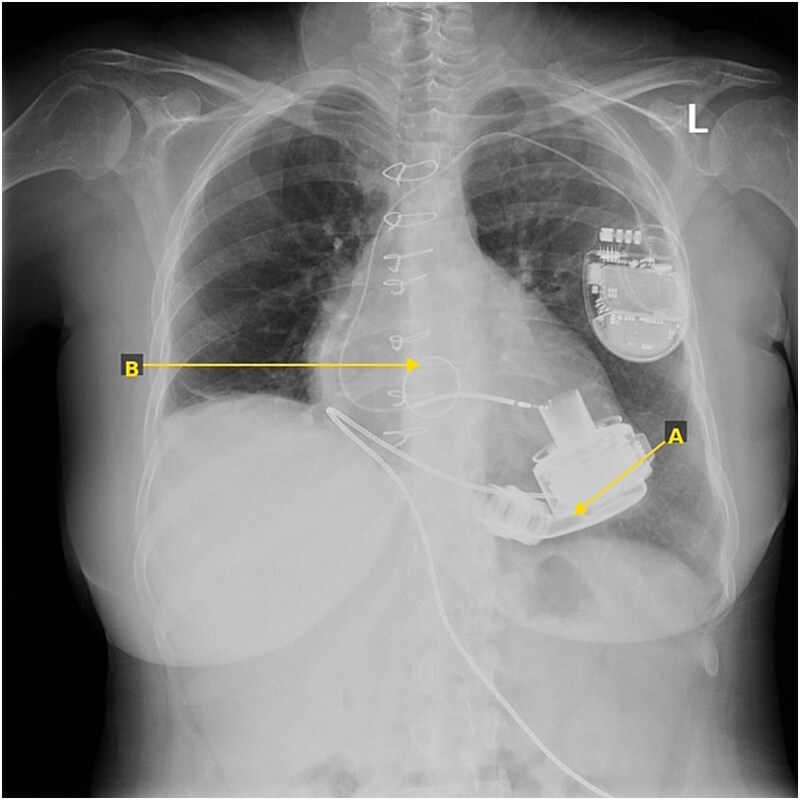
Postoperative posteroanterior chest radiograph demonstrating appropriate position of the HeartMate 3 pump, inflow cannula, and retained radiopaque mitral prosthetic sewing ring. The implantable cardioverter-defibrillator generator and transvenous leads are visible in the left pectoral region.
